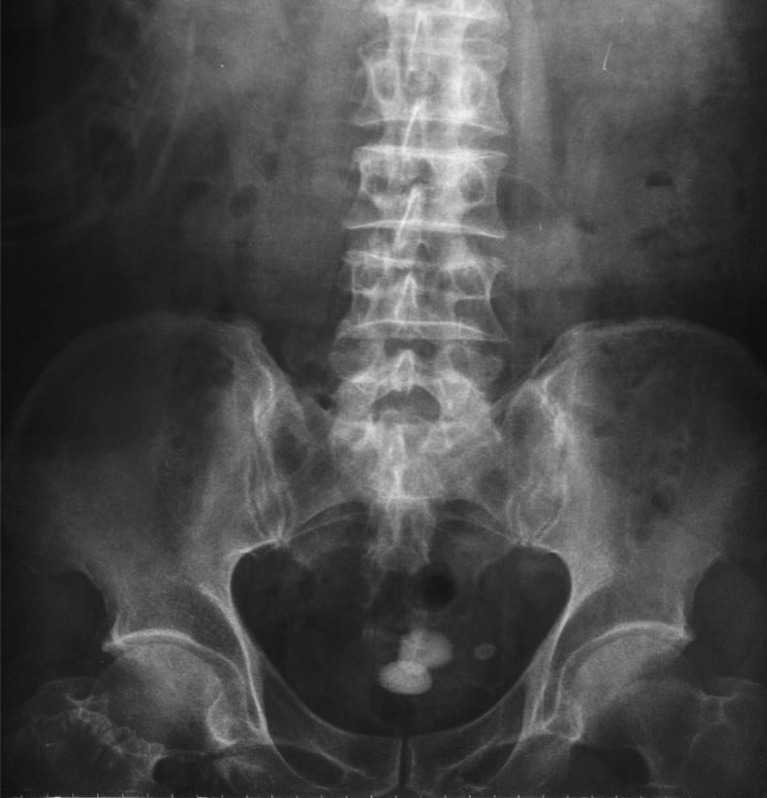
Preoperative KUB of a patient (case 1) with bladder diverticulum accompanied by bladder calculus. KUB, kidney, ureter and bladder X-ray.
